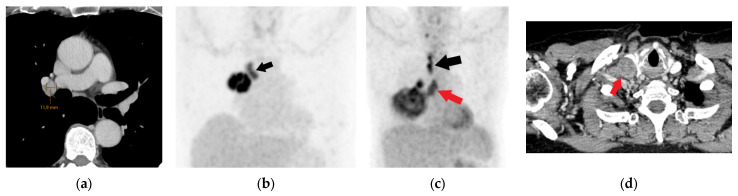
N Descriptors: Axial contrast-enhanced chest CT image showing metastasis in the ipsilateral hilar lymph node (arrow) or N1 disease (a). Maximum intensity projection (MIP) FDG PET image showing single-station N2a disease with metastasis in the ipsilateral lower paratracheal station (arrow, (b)). MIP FDG PET image in a patient with ipsilateral multiple-station (N2b) metastases in the ipsilateral lower paratracheal (black arrow) and subcarinal (red arrow) stations (c). Enlarged supraclavicular lymph nodes (arrow) indicate N3 disease (d).
